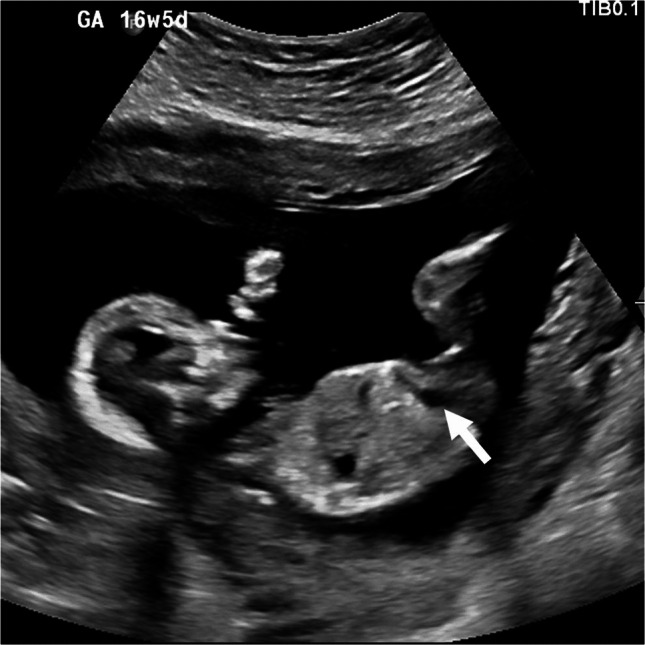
Sagittal ultrasound of a 16-week gestation male fetus showing the anechoic fetal urinary bladder (*arrow*)
